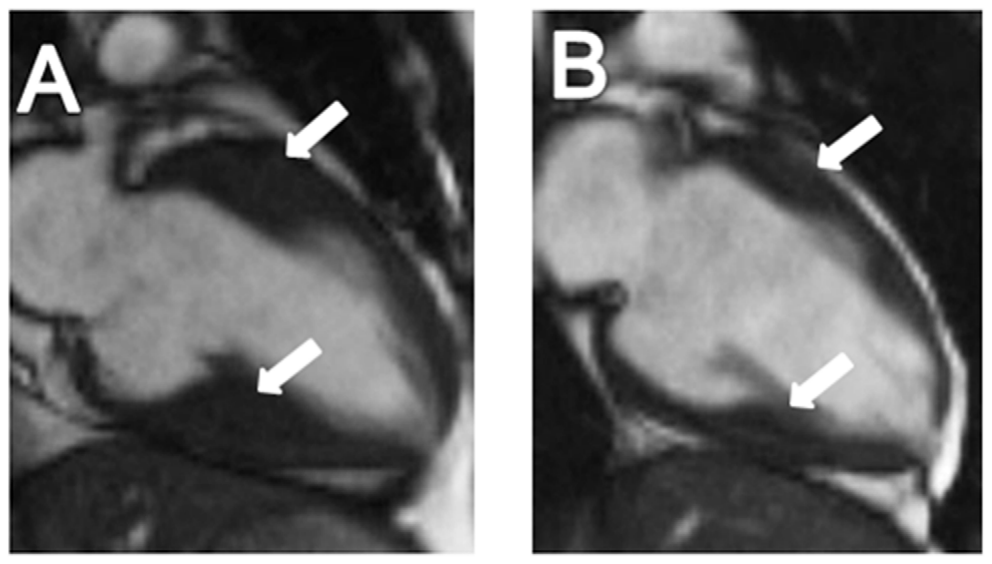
Cardiac magnetic resonance imaging (CMR) of the proband (III-2) (A) and his younger brother (III-3) (B). White arrows indicate areas of hypertrophy in the proband and asymmetrically hypertrophic and stiff ventricular wall in the younger brother (III-3).
